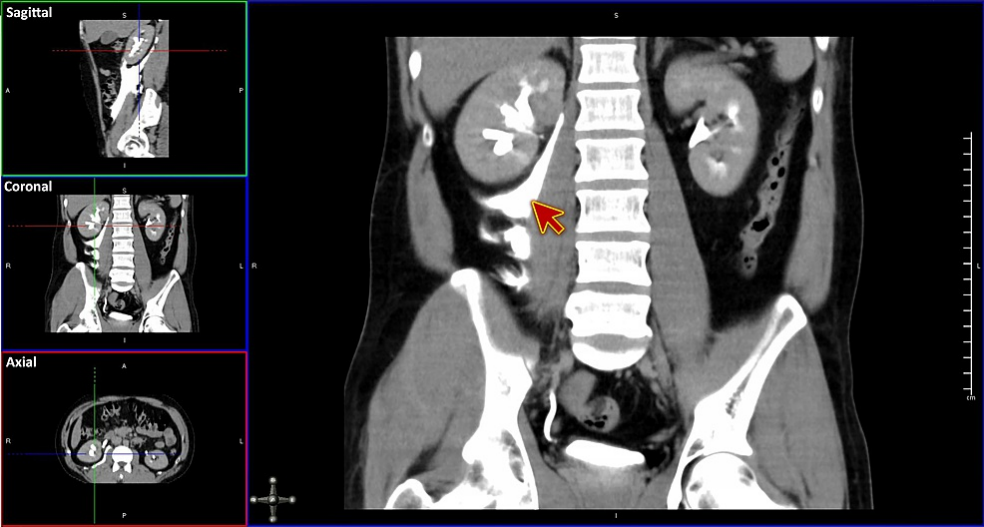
CT scan of the abdomen and pelvis with IV contrast showing perinephric and periureteric fat stranding with contrast extravasation (red arrow), going with urinoma. Multiplanar reformation or reconstruction (MPR) showing Sagittal, Coronal, and Axial planes
